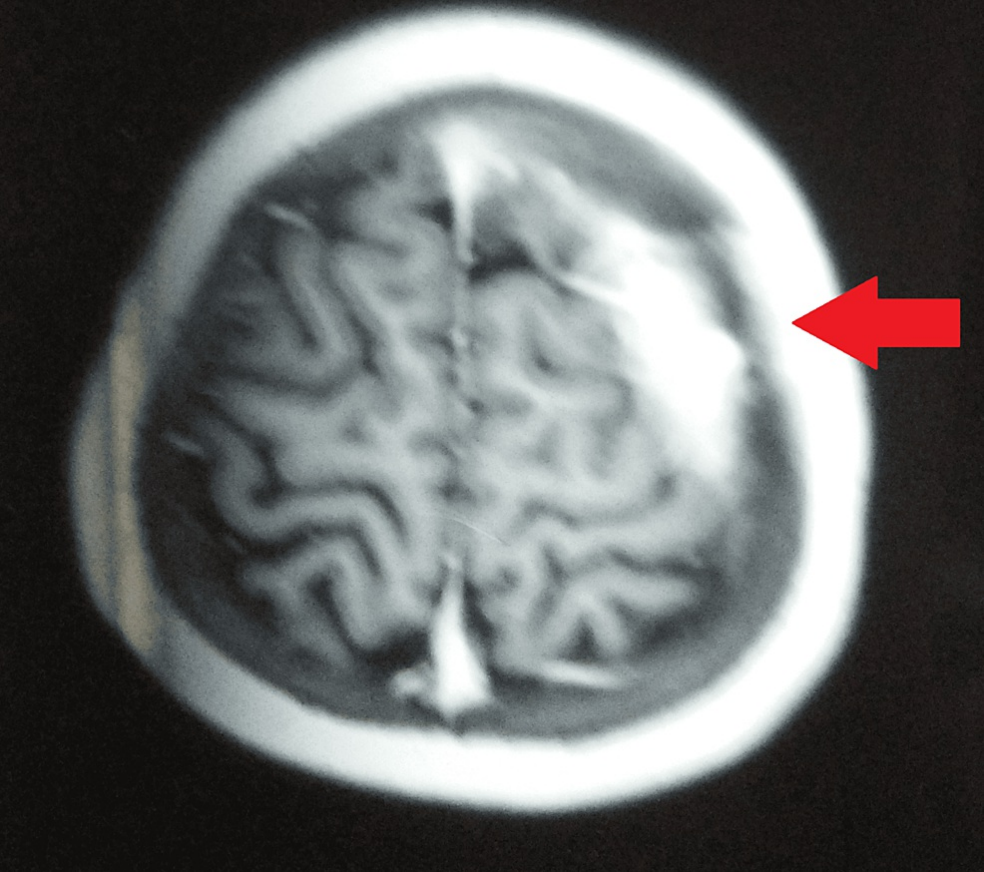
MRI showing the lesion situated on the left parietal bone and extradural abscess MRI: Magnetic resonance imaging
